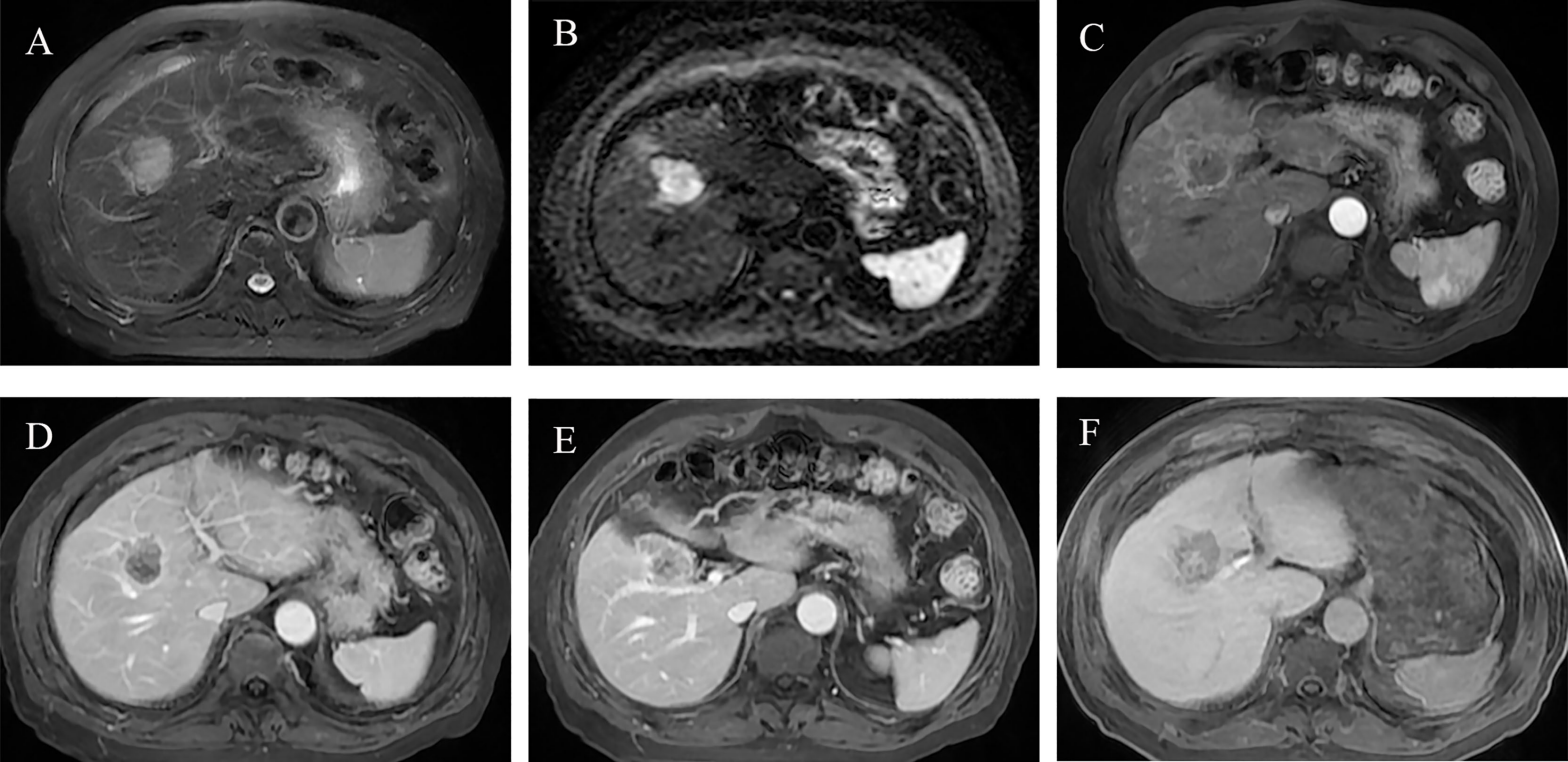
Preoperative MR images of early recurrence after anatomical hepatectomy in patients with hepatocellular carcinoma. A 72-year-old male with AFP-L3, PT, and GGT levels (negative, 10.7 s, 38 U/L) was hospitalized due to abdominal discomfort. Before operation, Gd-BOPTA MRI found a 3.7-cm lesion in hepatic segment V, with typical HCC features: mild hyperintensity on T2-weighted imaging (A), hyperintensity on diffusion-weighted imaging (B), and hypointensity on HBP (F). Rim arterial phase hyperenhancement present (C) and incomplete radiological capsule enhancement (E) were risk factors of early recurrence in our study. Arterial peritumoral enhancement, an independent risk factor for early recurrence, was presented on arterial phase and portal phase (C, D), white arrow). Peritumoral hypointensity on HBP (F, white arrow), also an independent risk factor. After anatomical hepatectomy, postoperative follow-up presented that this patient suffered from intrahepatic distant recurrence at 3 months.
